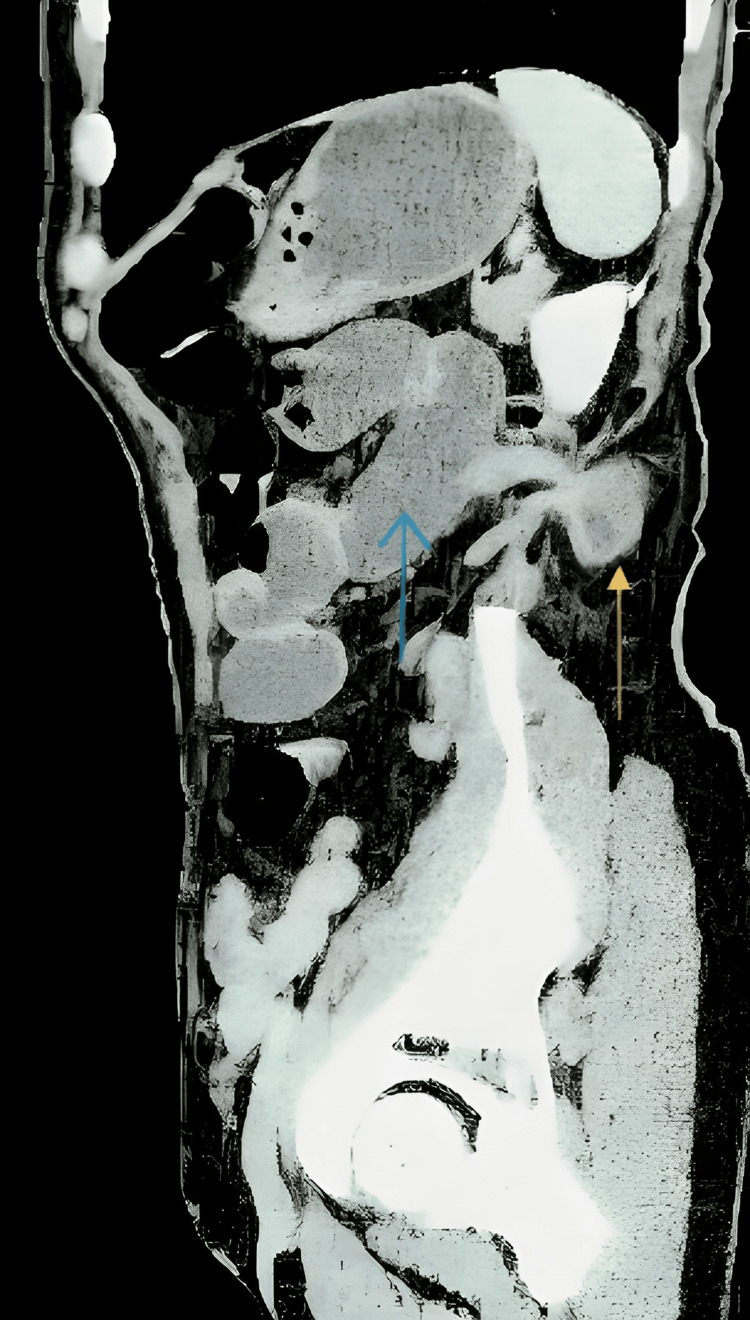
Sagittal CT scan section showing an incarcerated small bowel loop herniating through a superior lumbar fascial defect. Dilated bowel loop (yellow arrow) herniates through a 17 mm posterior lumbar fascial defect. Proximal bowel dilatation (blue arrow) indicates mechanical obstruction consistent with a Grynfeltt-Lesshaft hernia.
